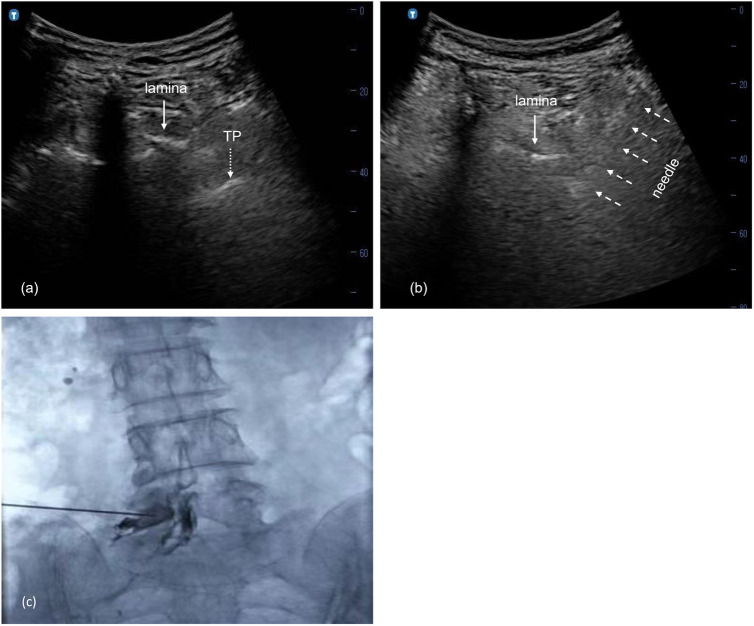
(a) Transverse view of US image at L5 intervertebral foramen; (b) selective nerve root (L5) block using the transforaminal approach under US guidance; the needle (dotted arrow) is advanced into the foramen using the in-plane technique; (c) contrast medium disperses into the L5 epidural and foraminal spaces in the fluoroscopic AP view. US, ultrasound; FL, fluoroscopy; AP, anterior–posterior; TP, transverse.
